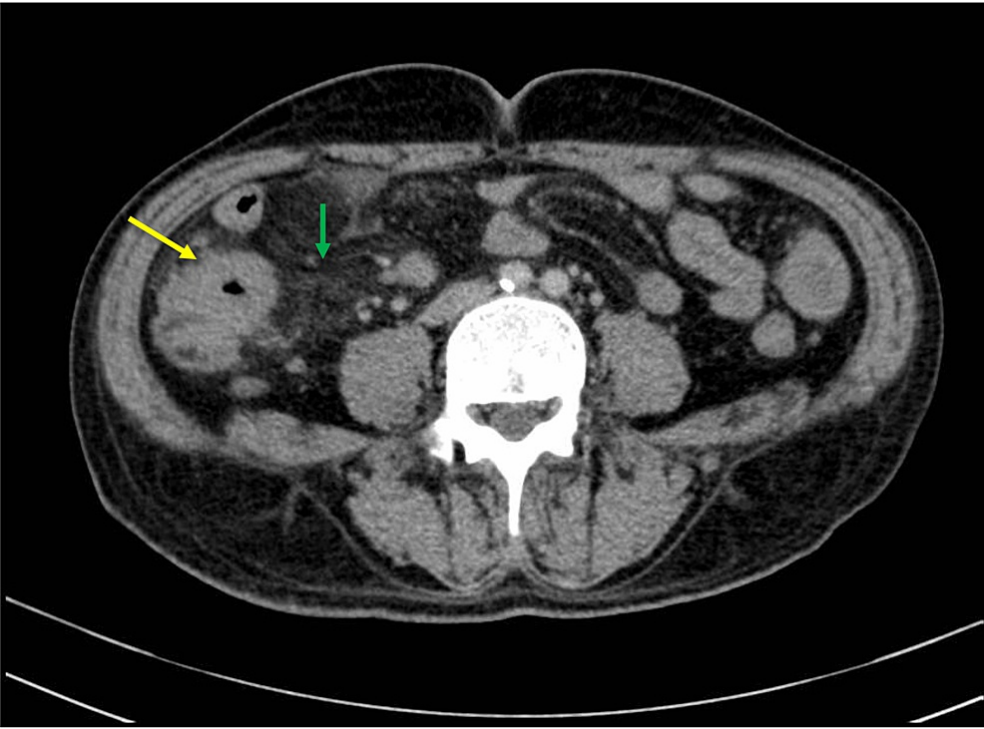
Contrast-enhanced abdominal-pelvic computed tomography. Thickening of the cecum (yellow arrow) and adjacent mesentery (green arrow).
